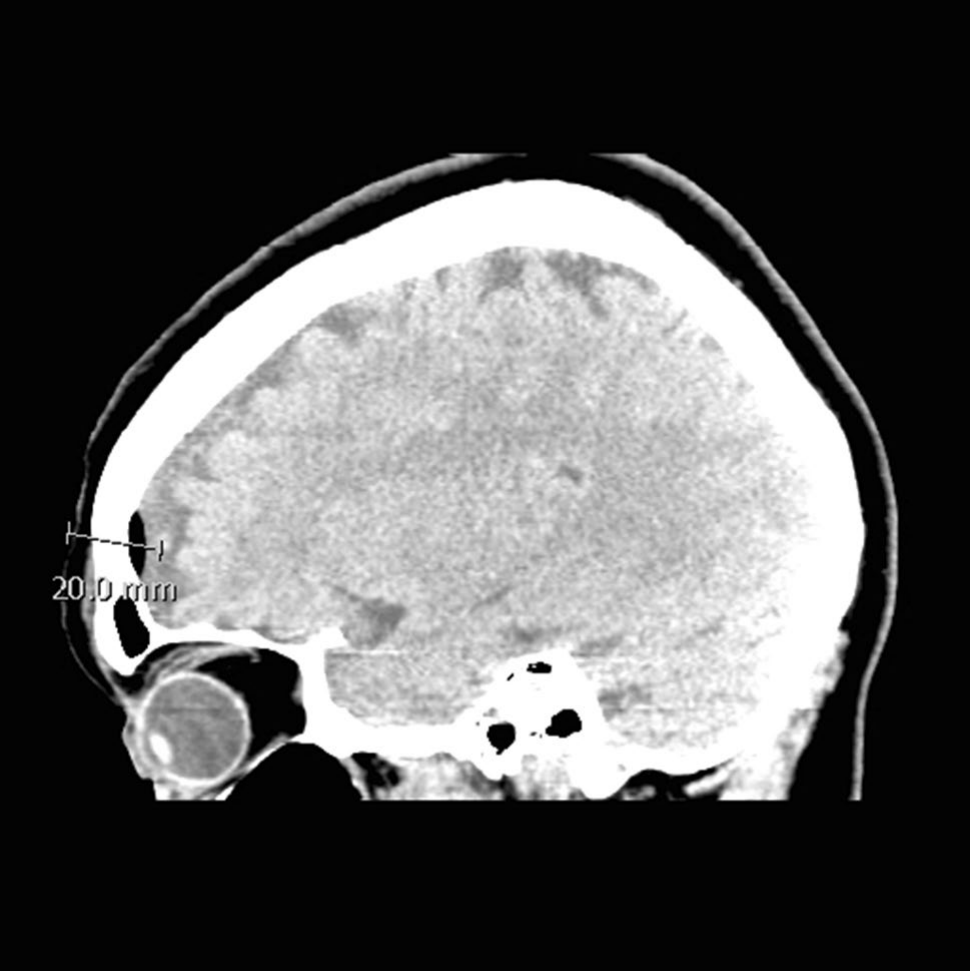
A representative postoperative sagittal CT scan. Distance from the skin to the cortical surface measured from the ocular papillary line 2 cm from the eyebrow
